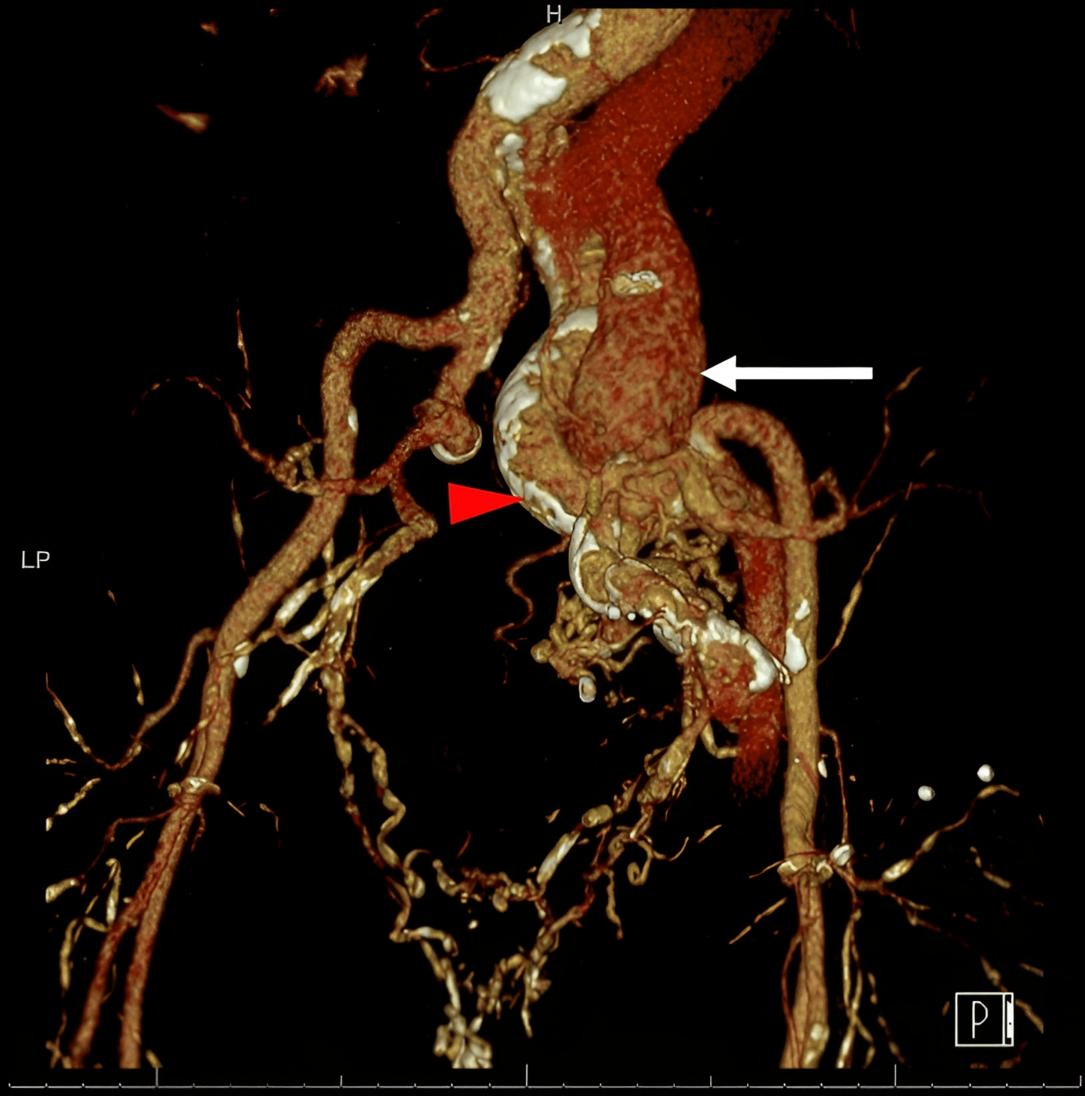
Three-dimensional CTA reconstruction of the arteriovenous fistula reveals marked calcification of the right internal iliac artery (IIA) (red arrowhead). The dilated right common iliac vein (CIV) (white arrow) is seen on this arterial phase due to early venous drainage, while the left CIV is not seen. Multiple overlapping dilated branches of the IIA are seen over the fistula. CTA: Computed tomography angiography. The 3D reconstruction using a Siemens Syndo, via the workstation at the Veterans Association (VA). All VA products are licensed (by paying a fee), and the reconstruction was done by Dr. Radu Serban.
